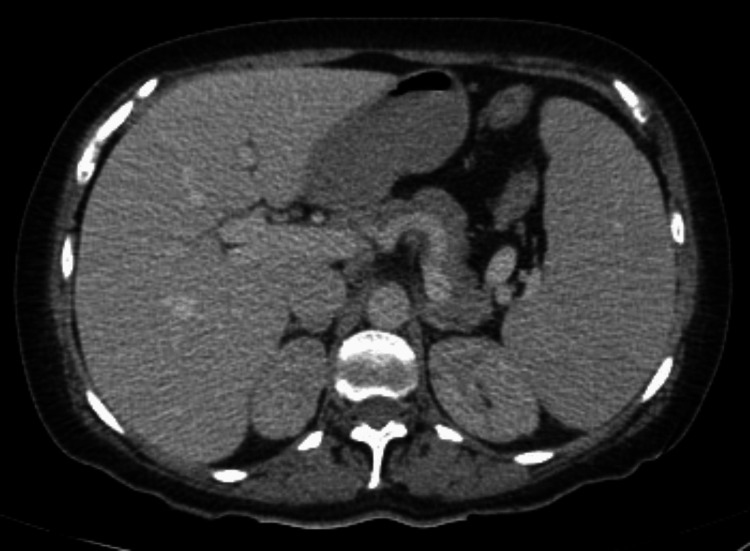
Contrast-enhanced abdominal CT scan in venous phase demonstrating hepatosplenomegaly, enlarged liver (21.4 x 17.5 cm) and spleen (17.1 x 15.2 cm)
